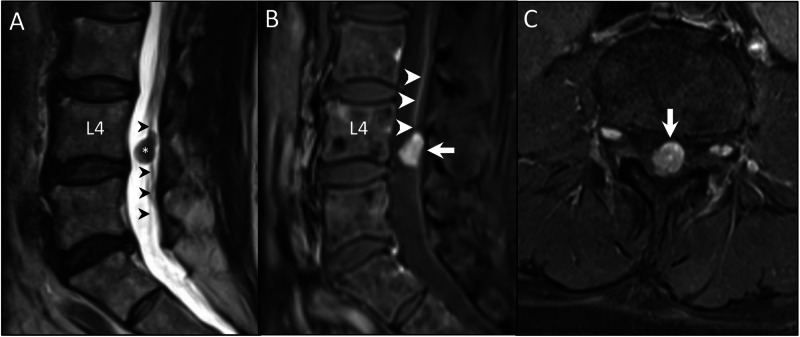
Preoperative MRI showing the tumor (A) T2 MRI revealing an isointense intradural-extramedullary lesion (asterisk) arising from and wrapped around the filum terminale internum (black arrowheads). (B and C) Sagittal and axial, respectively, T1 post-contrast MRI showing a well-demarcated and avidly enhancing (hypervascular) lesion (arrow) at the L4 vertebral level. Note the prominent vascular pedicle going along the cranial filum (white arrowheads).
